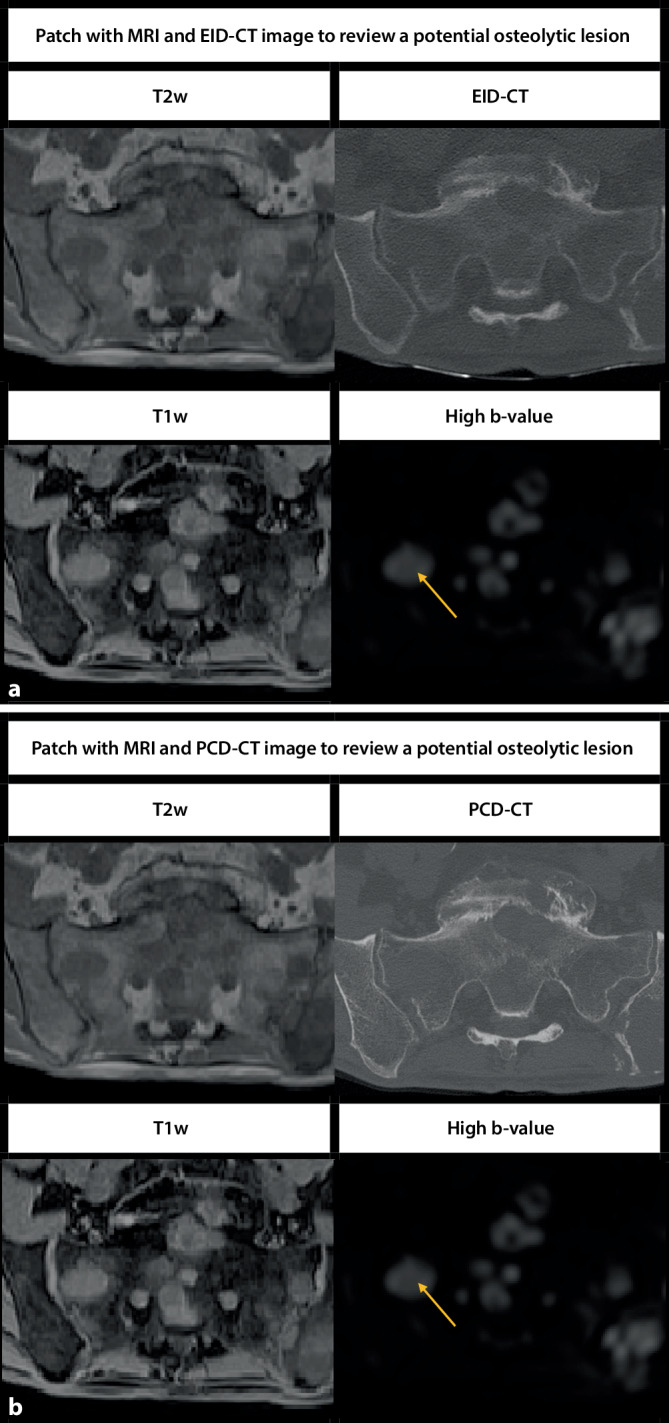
Visibility of lytic bone lesions in EID-CT (a) and PCD-CT (b). a *Upper patch* (2 × 2): axial T2-weighted image in the *upper left quadrant*, EID-CT slice in the upper right quadrant, T1-weighted image in the *lower left quadrant*, and DWI with high b‑value in the *lower right quadrant*. b *Lower patch* (2 × 2): axial T2-weighted sequence in the *upper left quadrant*, PCD-CT slice in the upper right quadrant, T1-weighted sequence in the *lower left quadrant*, and DWI with high b‑value in the *lower right quadrant*
